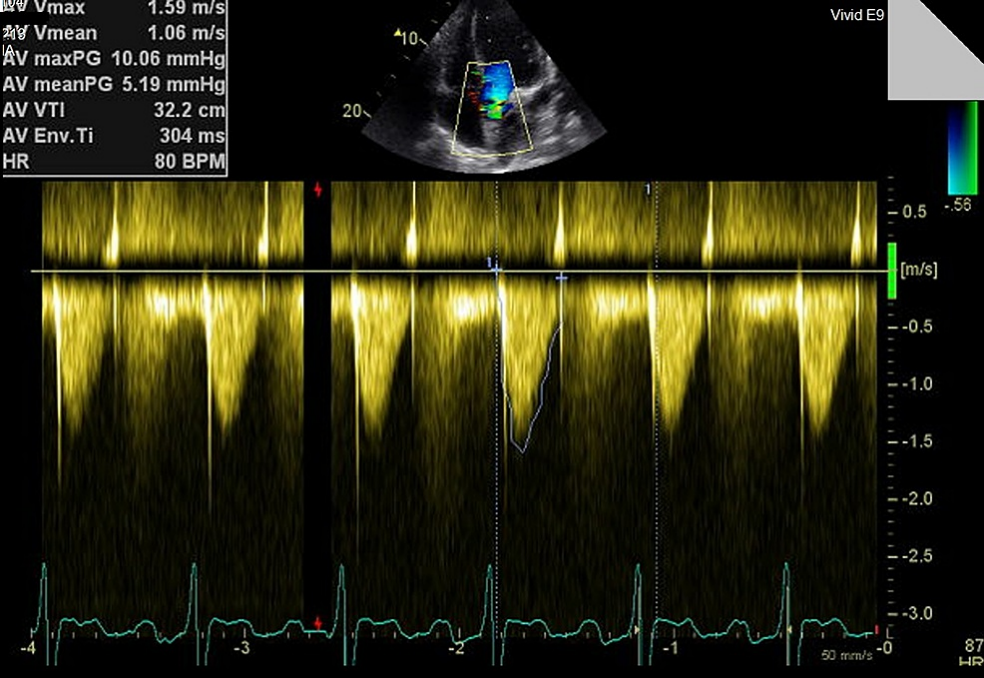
Continuous wave Doppler across the aortic valve showing normal velocity and normal mean gradient.
